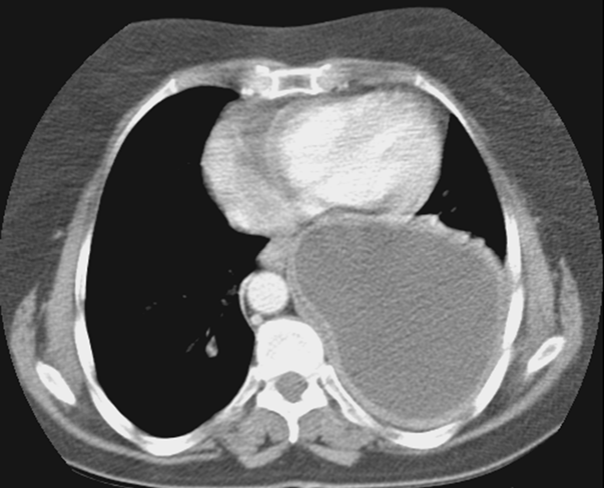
Contrast-enhanced computed tomography scan of thorax showing giant hydatid cyst of left lung.
